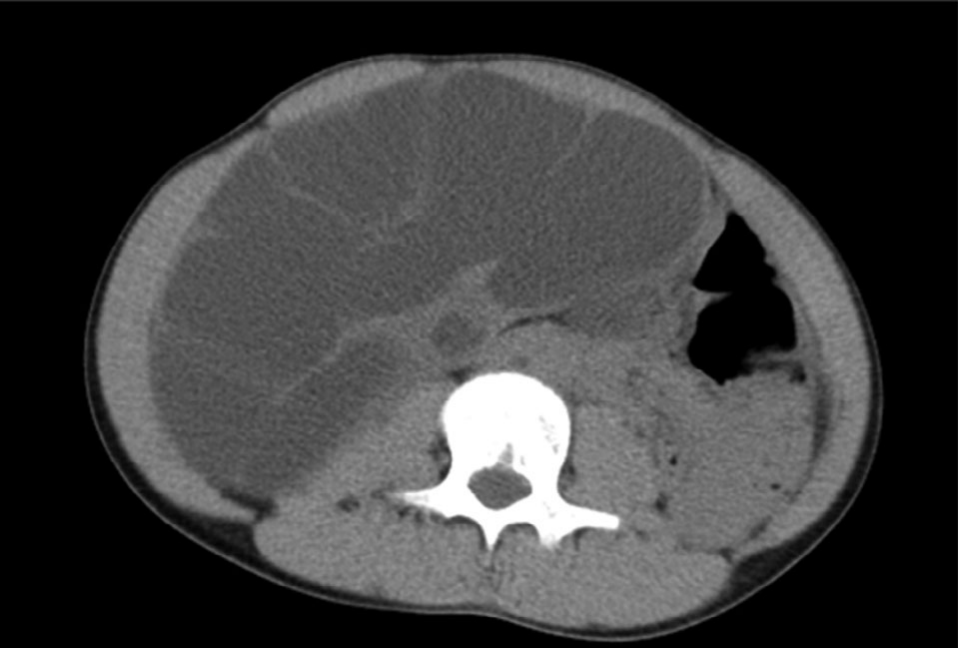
Abdominal CT performed during the first visit. The axial view reveals right hydronephrosis at the center of the body.
